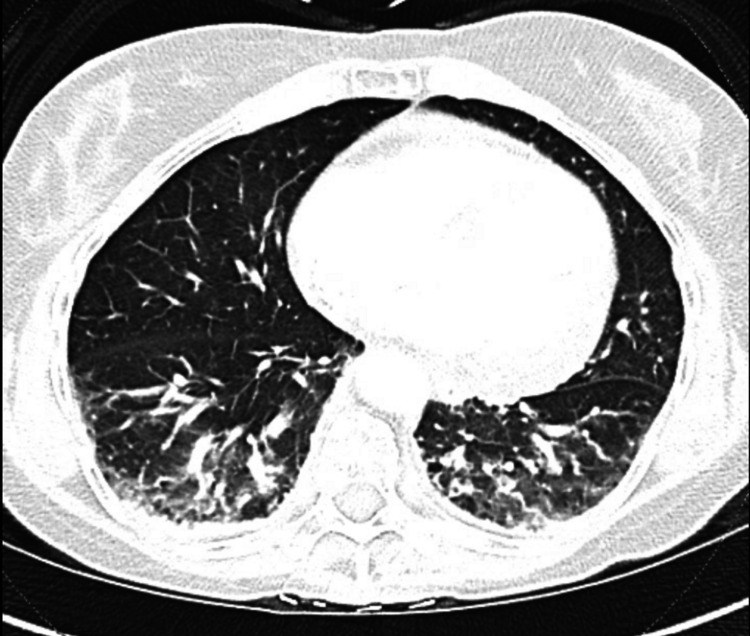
CT pulmonary angiography showing tree-in-bud nodularities CT: computed tomography
